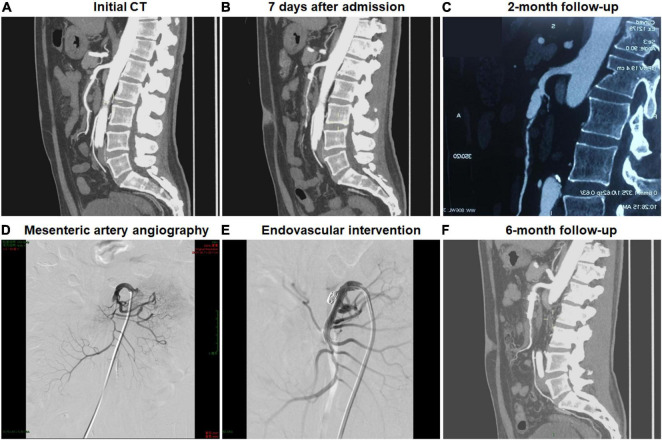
Endovascular coiling assisted stent placement and follow-up computed tomography (CT) for type III developed into type II spontaneous isolated superior mesenteric artery dissection. (A,B) Initial and 1-week CT angiography showed the thrombosed false lumen with ulcer-like projection in a 52-year-old man. (C) Two months later, type III lesion evolved into type II dissection with a patent “cul-de-sac” false lumen but no re-entry, and the true lumen was severely compressed. (D) Mesenteric artery angiography revealed the dissection formation and the occlusion of the main trunk. (E) Type II dissection was treated using stent placement and coil embolization *via* stent mesh. (F) Six month later, the stent remained patent.
